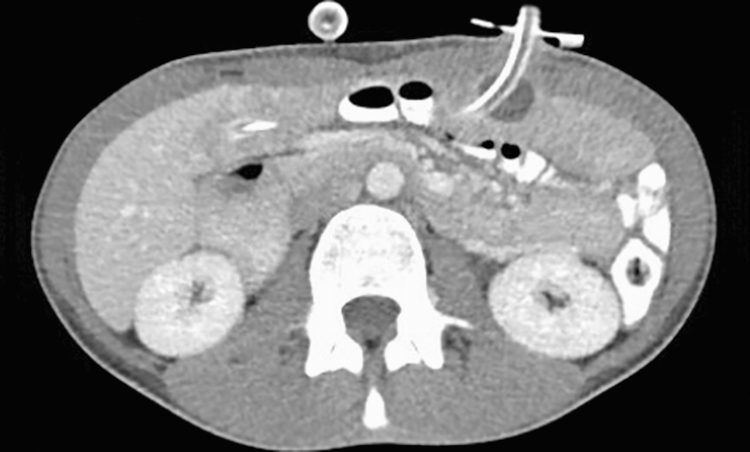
Axial computed tomography demonstrating resolution of small bowel obstruction following balloon deflation and gastric decompression.
